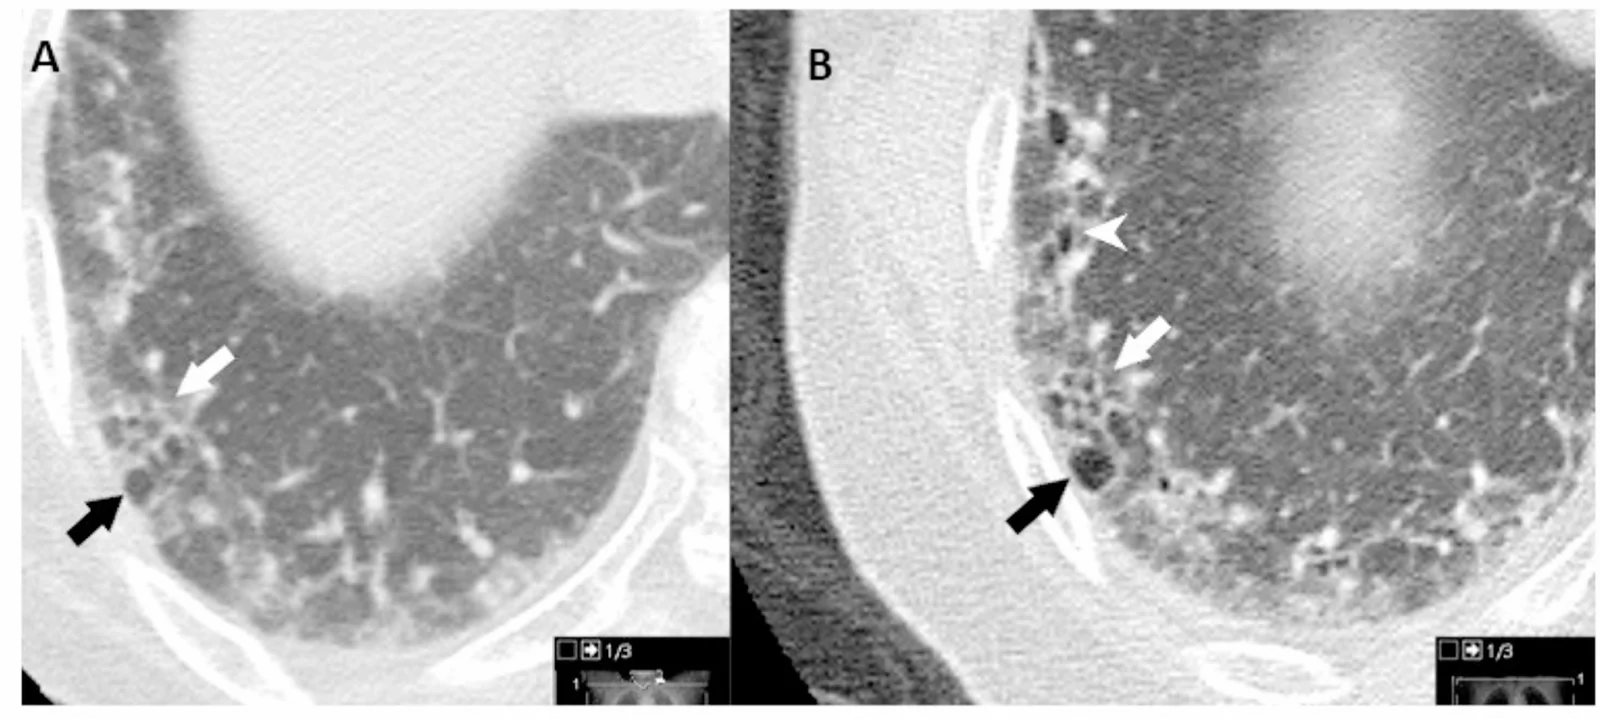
a and b: In a 86-year-old female patient with known rheumatoid arthritis, a UIP pattern is observed in computed tomography scans at 2-year intervals. High-resolution computed tomography image at the right pulmonary bases shows honeycombing seen as small, thick-walled cystic spaces arranged in multiple layers in the subpleural region which becomes more prominent in the later scans in figure b (white arrows). There is also a large subpleural cyst, which increases in size over time (black arrows). In figure b, traction bronchiectasis also shows progression (arrowhead)
